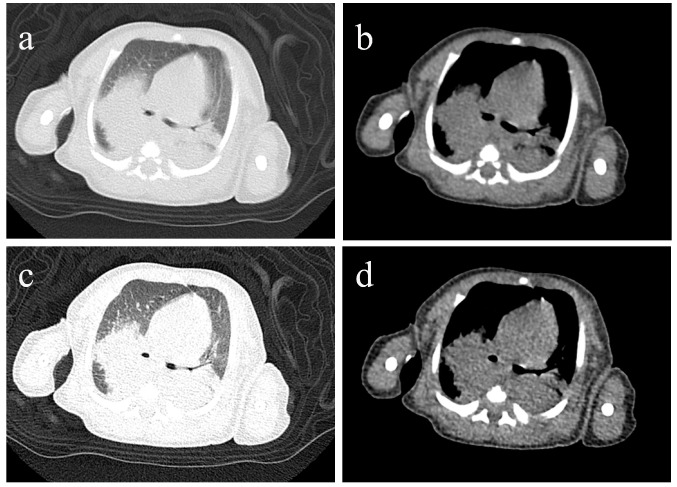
Chest CT scan of Case 1 prior to the first bronchoscopy (a–d). Images (a,c) are lung window settings, while images (b,d) are mediastinal window settings. They demonstrate increased bilateral lung markings, patchy opacities, and consolidation.
